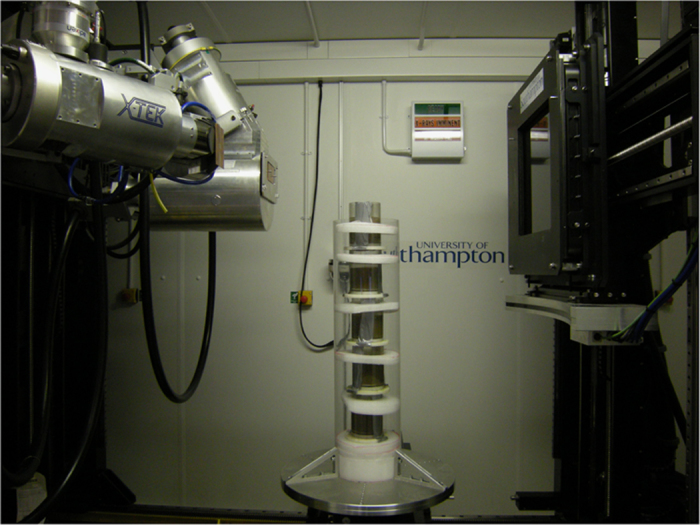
Five aquaria stacked in the holding brace in the micro-focus computed tomography scanner housed within the μ-VIS X-ray Imaging Centre, University of Southampton.
